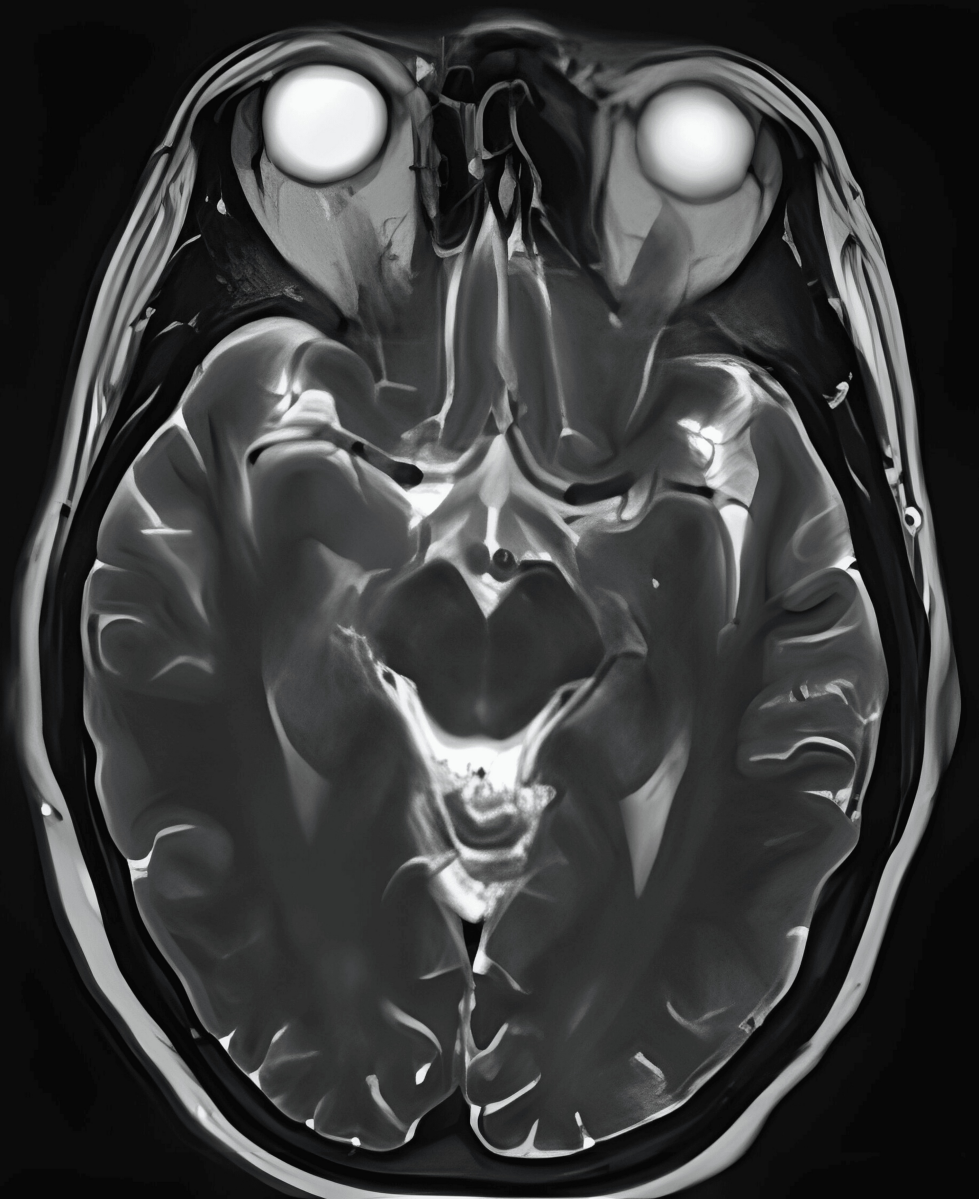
T2-weighted axial slice Resolution of multifocal areas of high intensity
